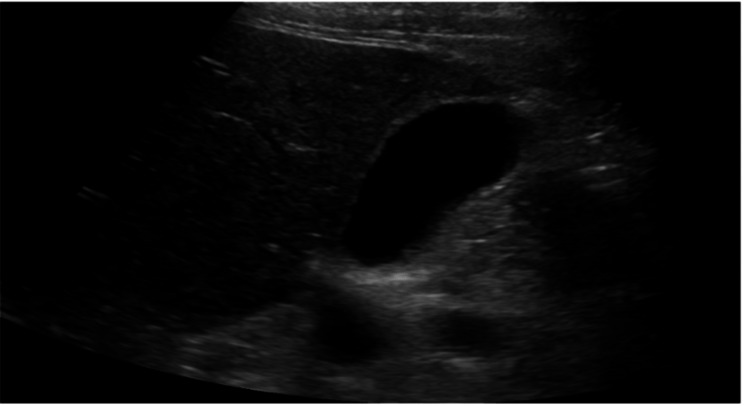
Right upper quadrant ultrasound revealing an unremarkable appearing gallbladder with no evidence of gallstones or wall thickening.
